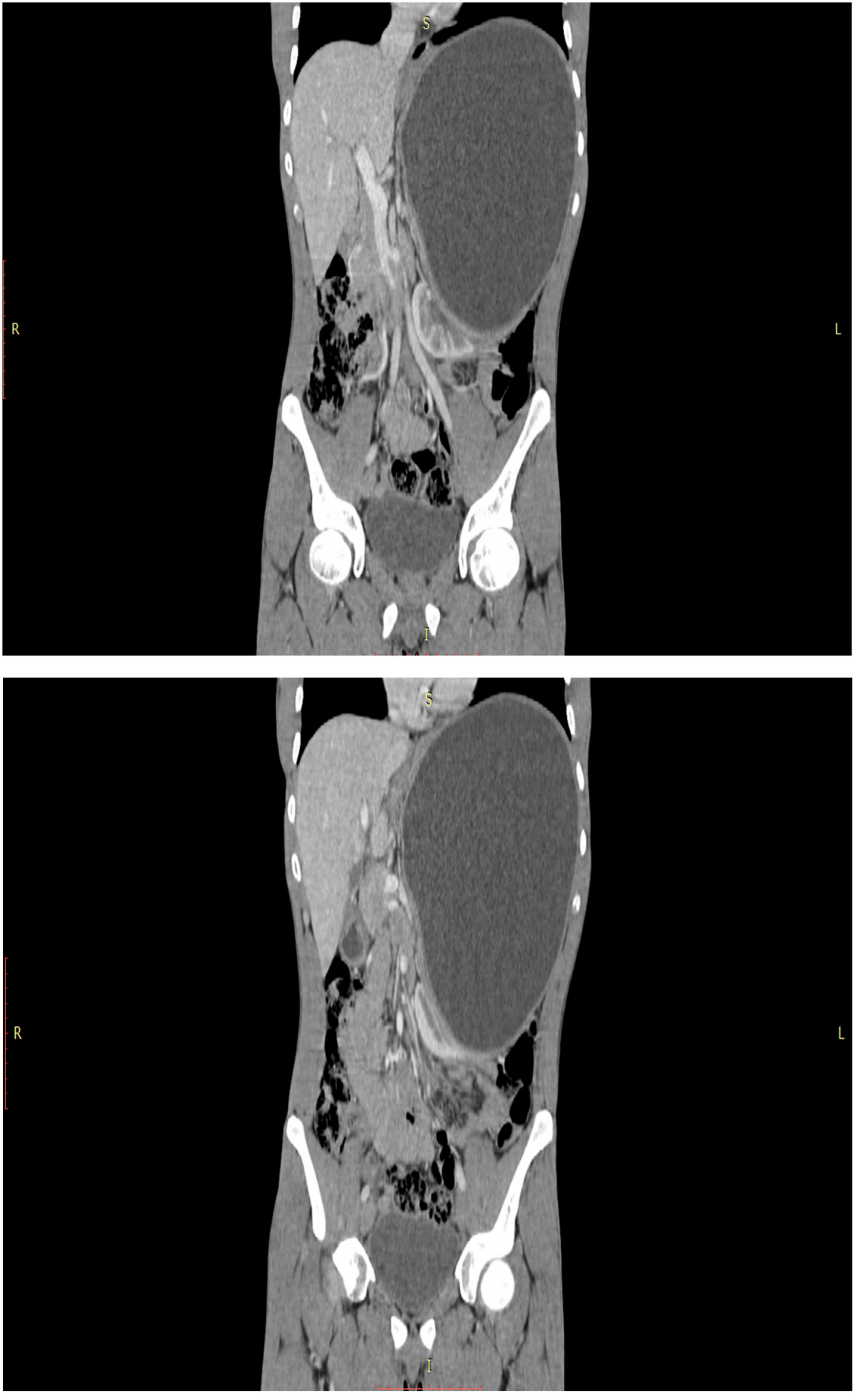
Coronal sections of abdomen CT scan without IV contrast
